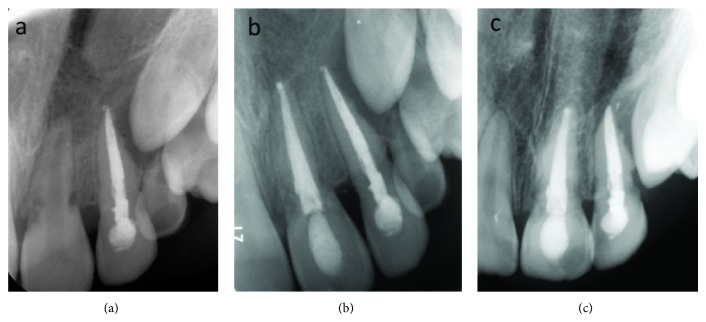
(a) Left upper central incisor with external root resorption at the 6-month follow-up, (b) periapical radiograph at the 12-month follow-up, and (c) periapical radiograph at the 24-month follow-up.
